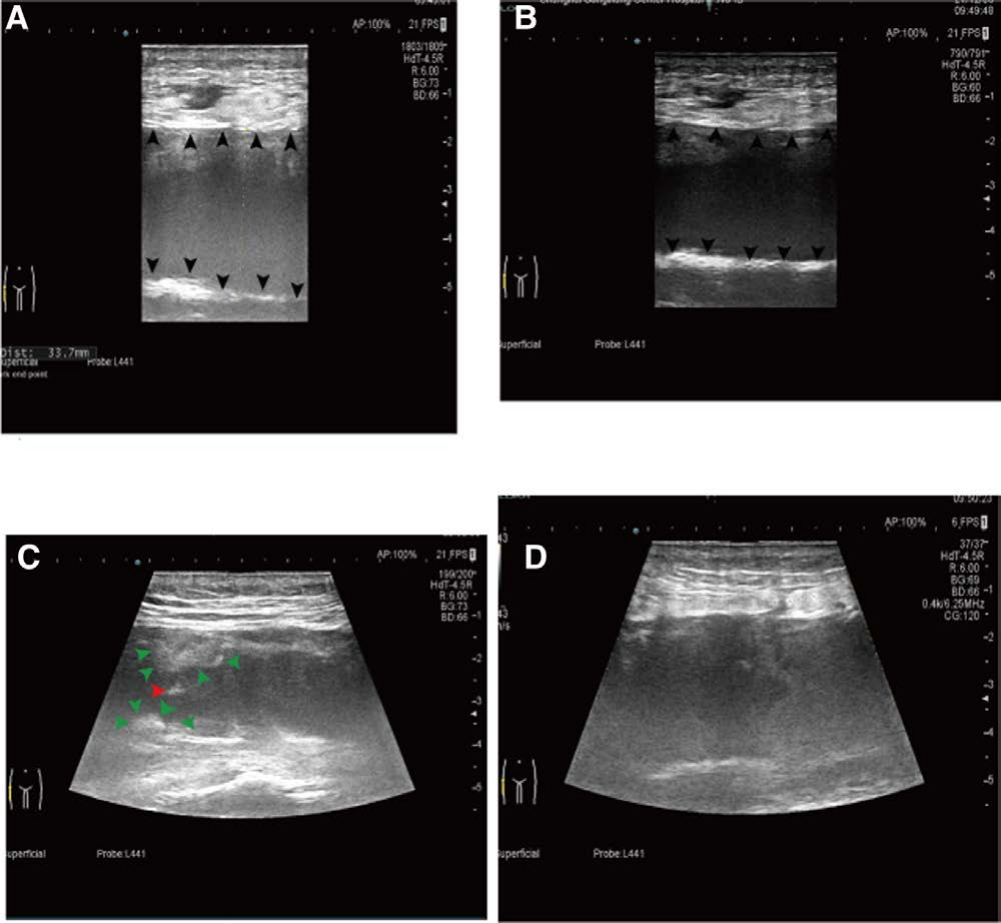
Ultrasound imaging of the right proximal femur reveals an inhomogeneous collection of mixed echogenicity. (A, B) The mixed echogenic collection is shown beneath the fat layer, with arrows demarcating its longitudinal upper and lower boundaries. (C) An irregular protuberance on the inner surface of the mixed collection is represented in green, while a potentially highly echogenic fat particle is denoted in red. Color Doppler flow imaging. (D) CDFI did not manifest any discernible blood flow signal. CDFI = color Doppler flow imaging.
